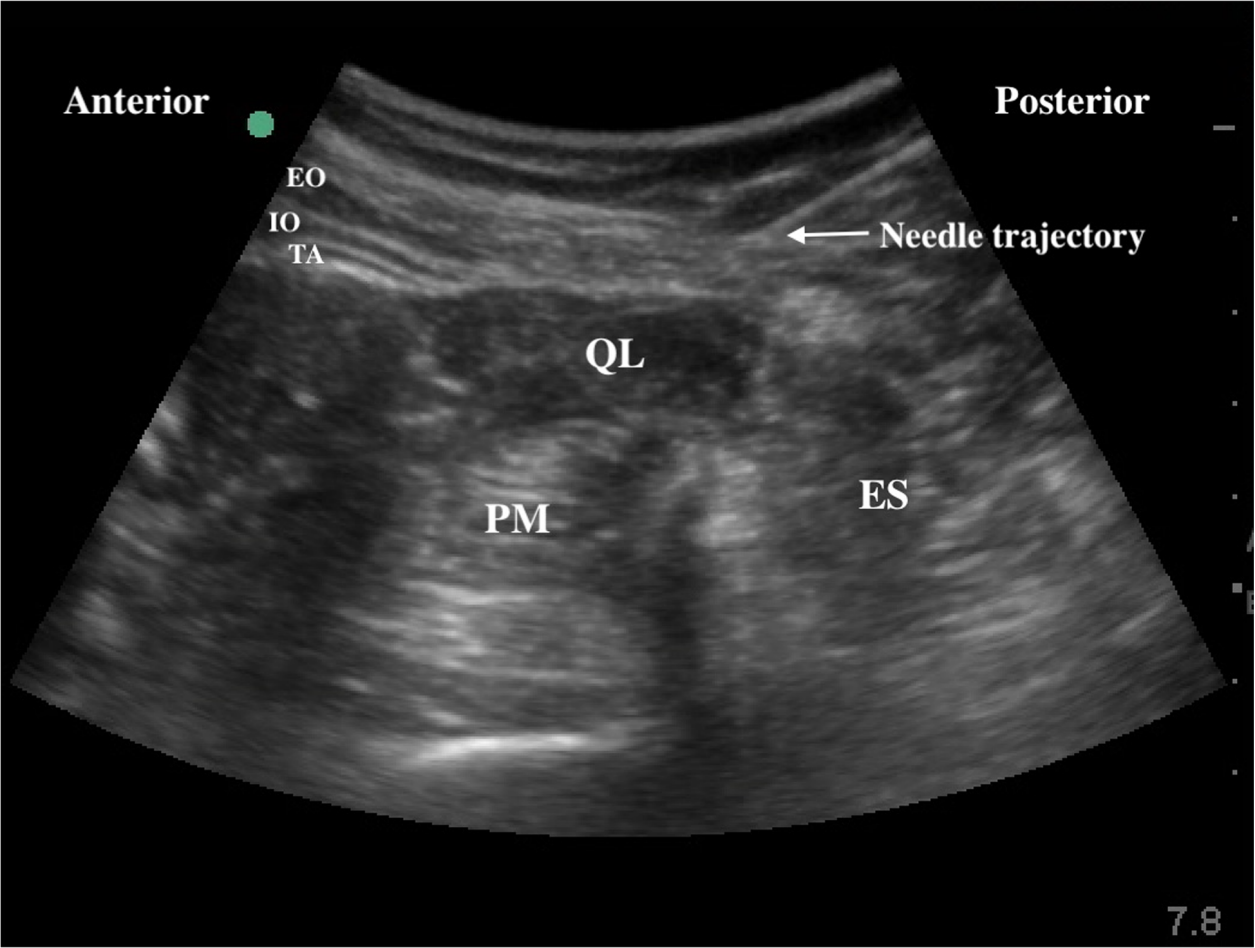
Ultrasonographic image of QLB. Arrow shows the direction of needle. EO, external oblique muscle; IO, internal oblique muscle; TA, transversus abdominis; QL, quadratus lumborum; PM, psoas major; ES, erector spinae
